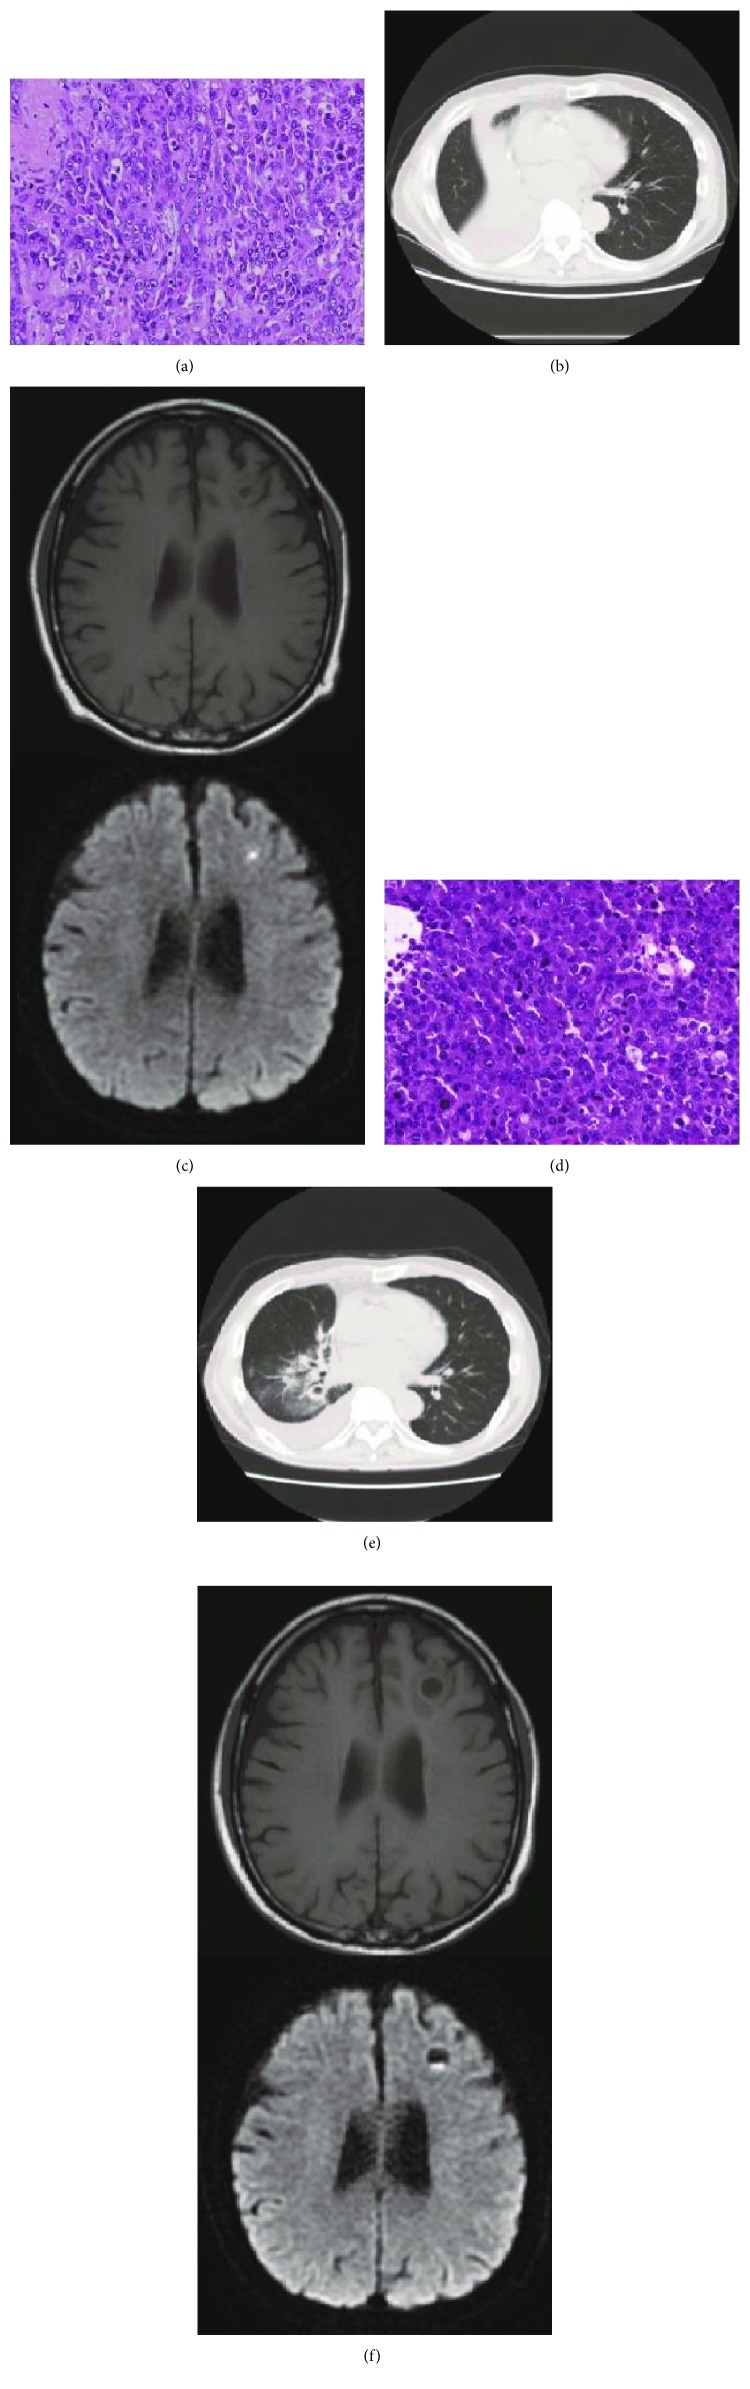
(a) Pathological findings: lung cancer tissue after pulmonary resection (×400 at original magnification). (b) Chest CT taken at admission revealed consolidation and atelectasis in the right lower lobe and right pleural effusion. (c) A 5 mm cranial lesion was found by head MRI at admission (upper panel: T1-weighted MRI, lower panel: diffusion-weighted MRI). (d) Pathological findings: the brain lesion which was resected (×400 at original magnification). (e) Chest CT taken three weeks later showed that consolidation and atelectasis improved, revealing ground glass opacities and multiple cavitary lesions in the right lower lobe. (f) On day 27, the brain lesion increased to 14 mm and exacerbation of cerebral edema around the brain lesion was observed by head MRI (upper panel: T1-weighted MRI, lower panel: diffusion-weighted MRI).
